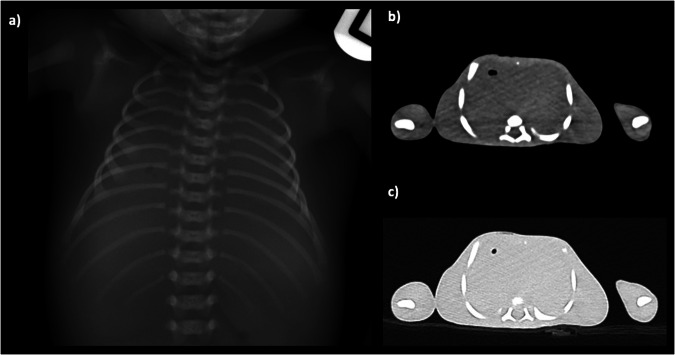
Postmortem chest radiograph (a) and axial PMCT images of the lungs in soft tissue (b) and lung windows (c) in a newborn term baby. There is minimal soft tissue contrast differentiation making cardiac and lung imaging nondiagnostic
